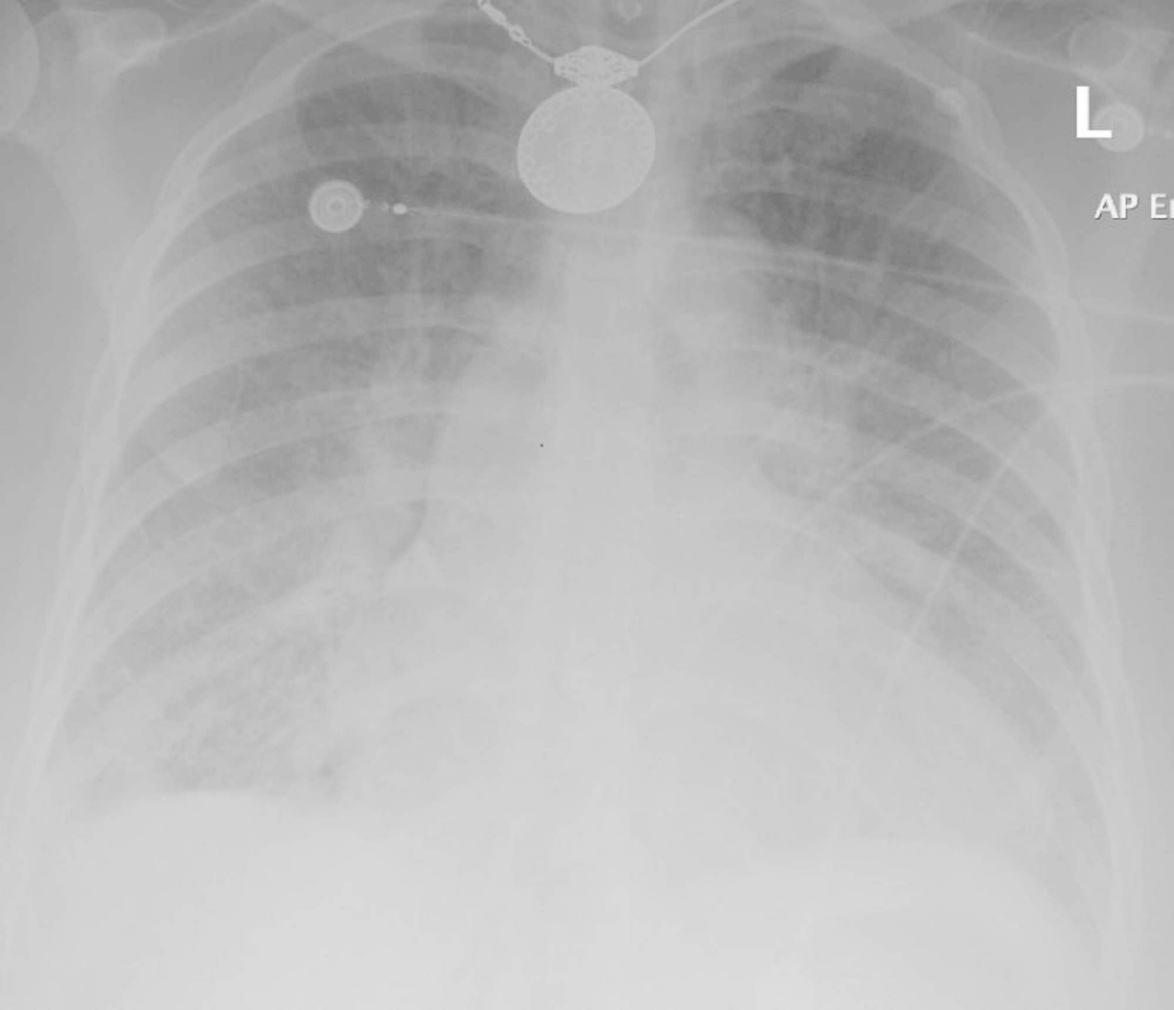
Chest X-ray showing pulmonary edema
